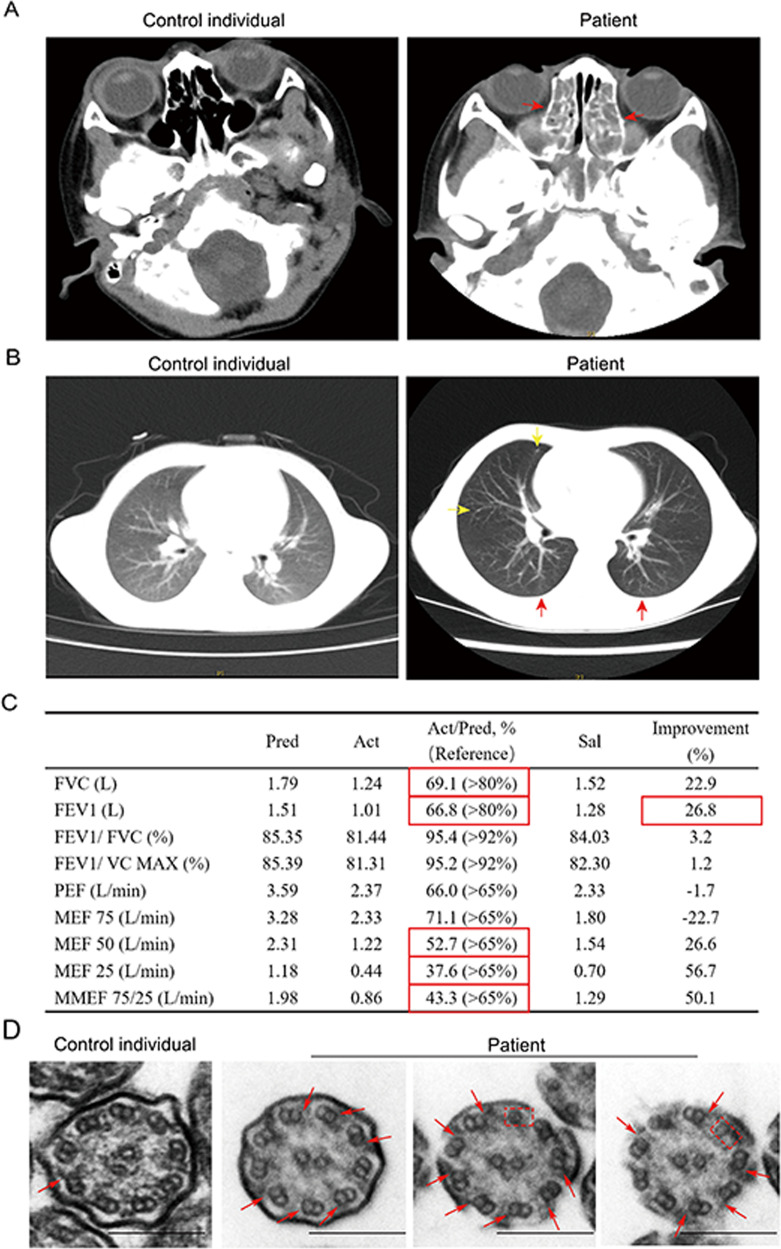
The patient with PCD shows reduced lung function and abnormal “9 + 2” structure. A CT scan analysis of the nasal sinuses shows pansinusitis in the patient. The red arrow reflects nasosinusitis. B Chest plain image of the patient by CT scan showing the bronchiectasis in the patient. The red arrow reflects pulmonary interstitial changes; the yellow arrow reflects small nodules. C The results of spirometry in the patient with PCD demonstrating inspiratory airflow limitation and compromised lung function. Pred: predicted value; Act: actual measured value; Act/Pred: ratio of actual measured to predicted values; Sal: measured value after inhaling salbutamol; Improvement: ratio of Sal/Pred to Act/Pred; FVC: forced vital capacity; FEV1: forced expiratory volume in one second; FEV1/FVC: ratio of FEV1 to FVC; FEV1/VC MAX: ratio of FEV1 to VC MAX; PEF: peak expiratory flow rate; MEF 75: maximal expiratory flow after 75% of the FVC has not been exhaled; MEF 50: maximal expiratory flow after 50% of the FVC has not been exhaled; MEF 25: maximal expiratory flow after 25% of the FVC has not been exhaled; MMEF 75/25: maximal mid-expiratory flow. The measurement of FVC and FEV1 are 69.1% and 66.8%, respectively, suggesting restricted ventilation dysfunction. The MEF 50 and 25 were less than 65% suggesting small airway airflow obstruction. After inhaling salbutamol, the FEV1 improved to 26.8%, suggesting positive bronchial diastolic test. Reference range is shown in bracket; red boxes indicate abnormal value. D Ultrastructure of the ciliary axonemes from normal child and the patient by TEM showing the ODAs defects of ciliary axonemes from the patient. The red arrows indicate the ODA structure in control individual and the lack of ODA in the patient. The red dash box indicates single microtube existing in the patient. Scale bar = 200 nm.
